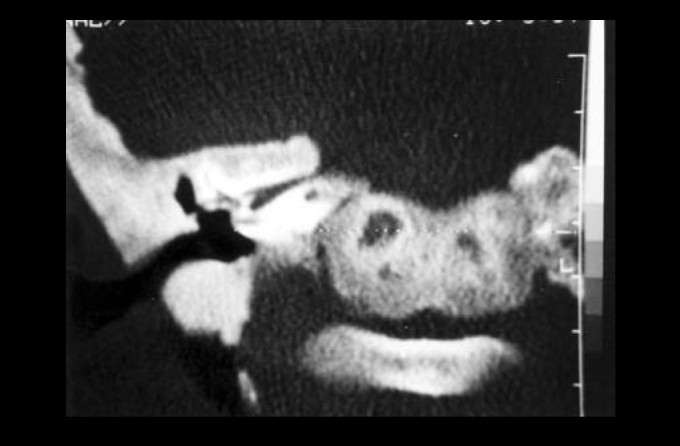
Case 3: Temporal bone CT scan (coronal section) in patient with Camurati-Engelmann disease, evidencing impairment of temporal bone, bone compression of internal acoustic canal on the right.
